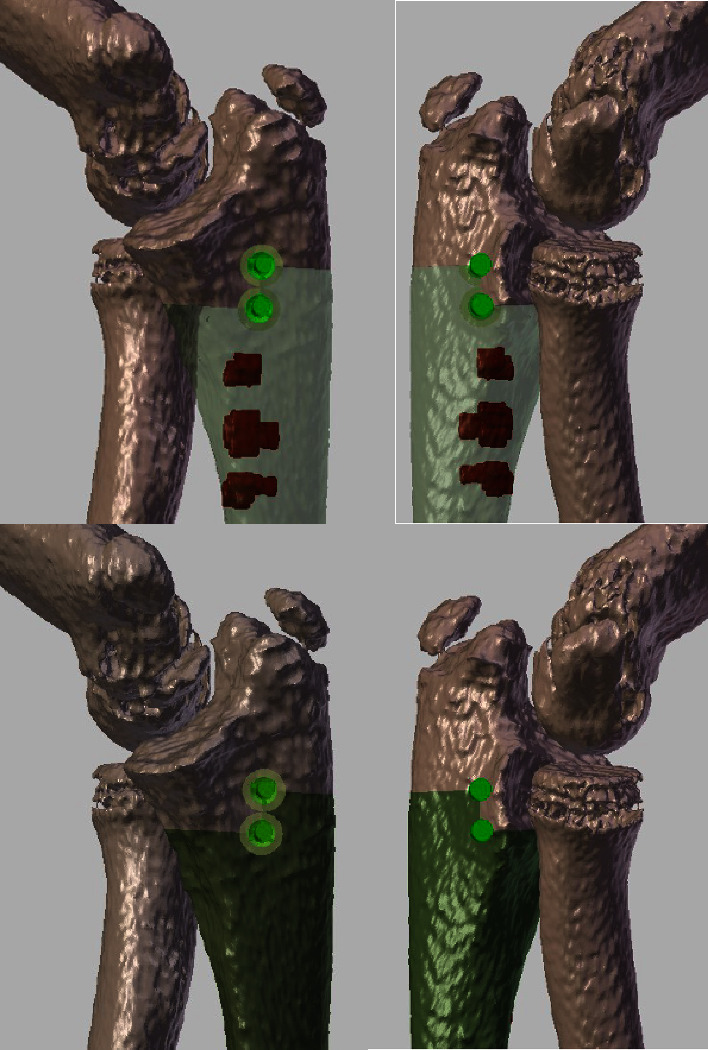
The same patient mentioned in Figure 1. Top left and right: the segmented tumor on MRI was merged with the CT scan. The tumor is in red (there were 3 small separated tumoral areas in the proximal ulna). The picture gives the detail of the preoperative planning for the proximal section. A step cut was chosen to improve stability. The chosen margin was 5 mm in order to preserve the joint. Bottom: the green zone in the ulna is the area that is planned to be resectioned. Left: medial view and right: lateral view.
